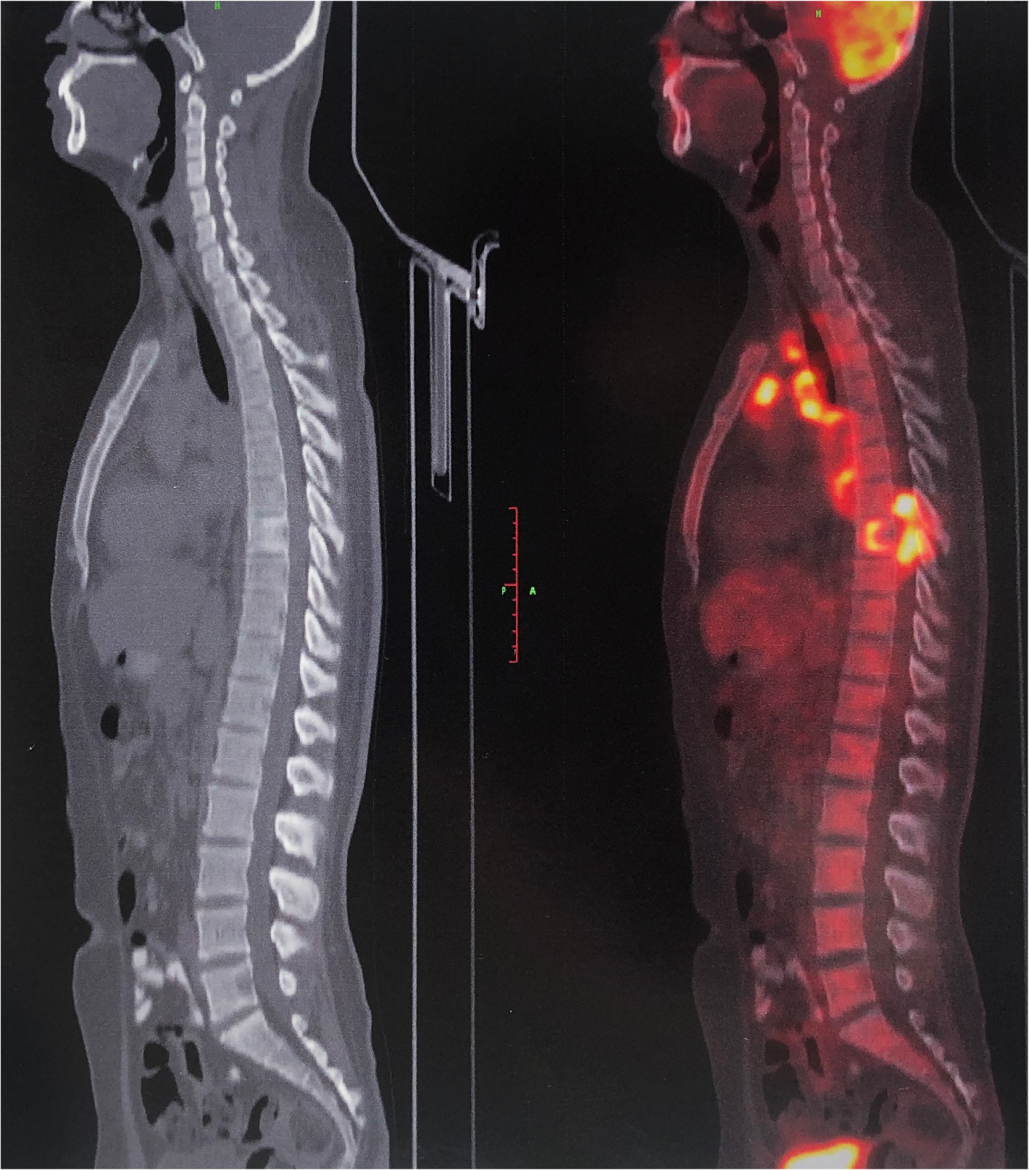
Maximum intensity projection image of PET/CT showing the high metastasis in ileocecal intestinal and terminal ileum, multiple LNs, bones and soft tissue
